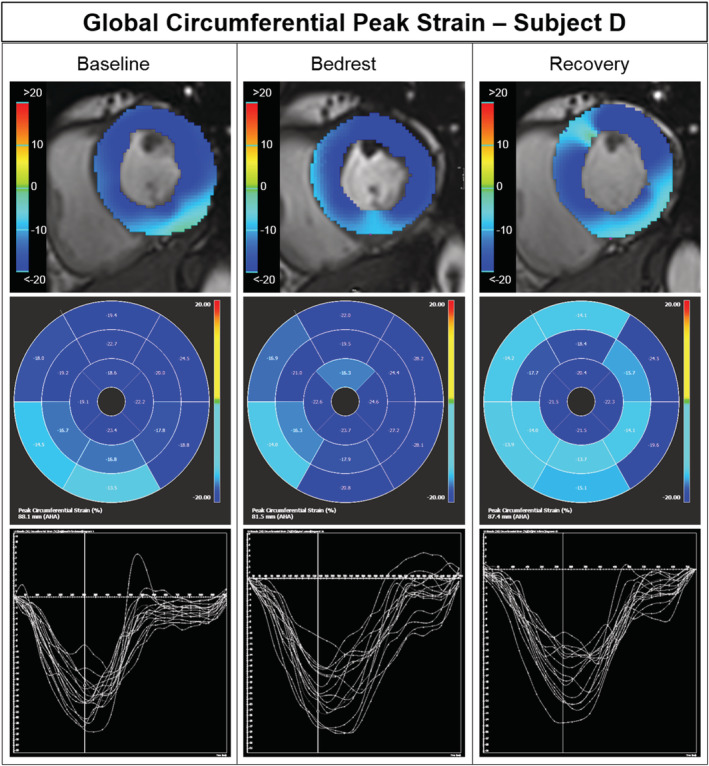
Global circumferential peak strain. Circumferential peak strain measurements at baseline, end of bed rest, and end of recovery in a representative study participant. Upper panel: end‐systolic cross‐sectional short‐axis cardiac magnetic resonance imaging images at the level just above the papillary muscles with circumferential strain overlay. Middle panel: Bull's eye view of the 16 American Heart Association (AHA) myocardial segments model with circumferential peak strain values and colour coding, where deeper blue resembles higher strain values. Lower panel: Circumferential peak strain time course over one heartbeat for the 16 AHA myocardial segments model.
